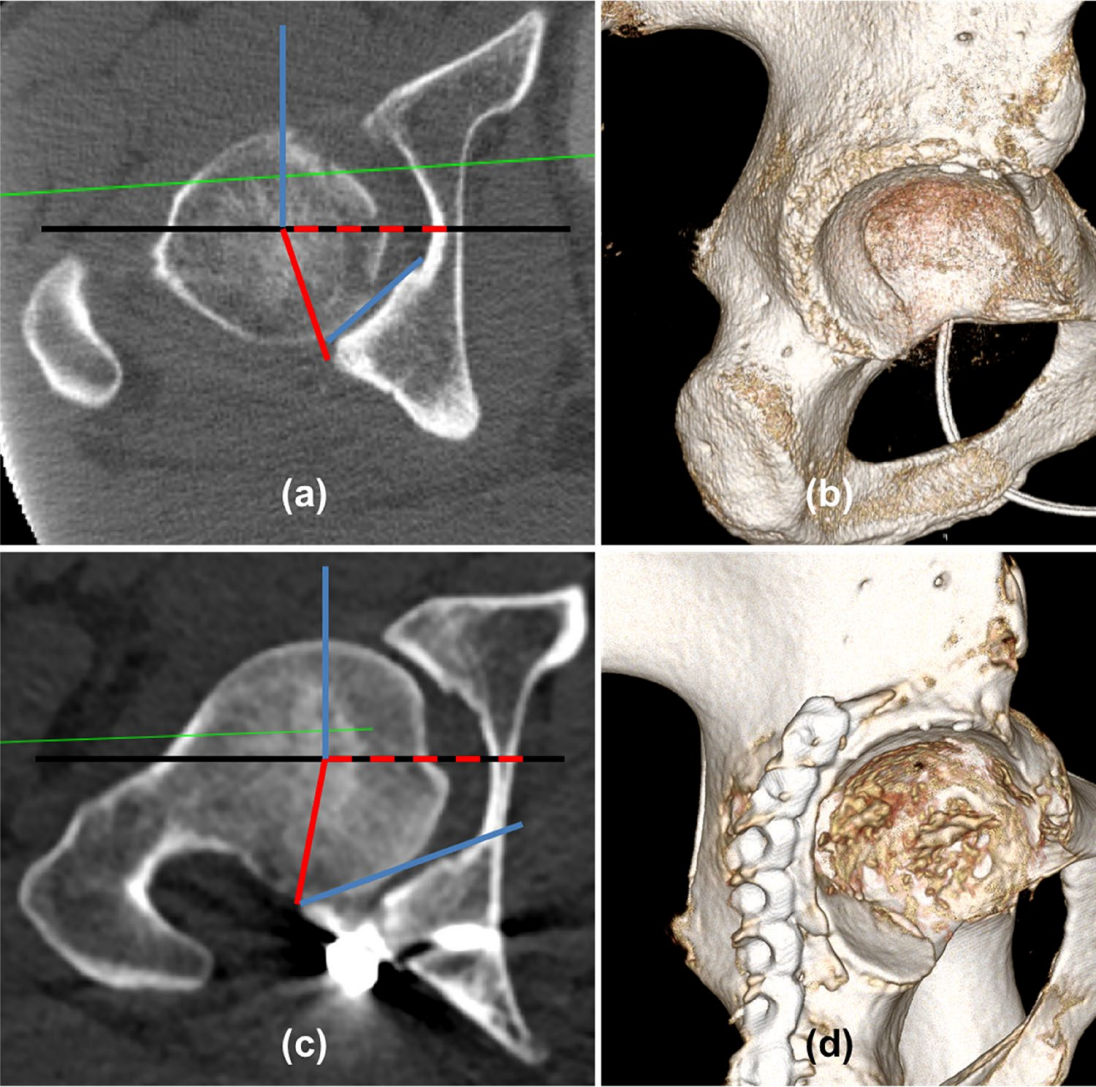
CT-morphological acetabular measurement. a and b A 35-year-old man with traumatic posterior hip dislocation with femoral head fracture but without posterior wall fracture. c and d A 34-year-old patient with a traumatic posterior hip dislocation without femoral head fracture but with posterior wall fracture. a and c Strictly axial images of the right hip. b and d Strict lateral view on 3D reconstructions of CT scans showing a small and open acetabulum in image b and a large and closed posterior wall in image d. Red dashed line (ICL): line between both femoral head centers, black line: extension of the ICL, blue lines: line perpendicular to the ICL defined as ICL90 and line defined as tangent to the posterior acetabular joint surface. Posterior acetabular sector angle (PASA): angle between both red lines, posterior wall angle (PWA): Angle between both blue lines
